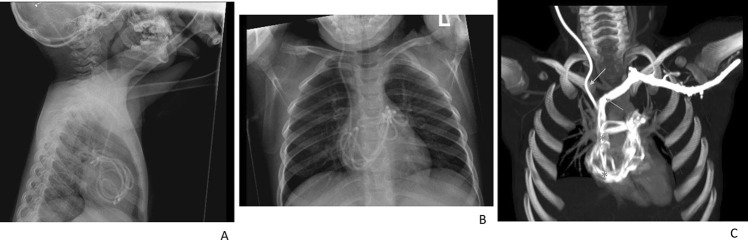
A, B Presenting lateral and AP chest X-rays demonstrating intracardiac positioning and coiling of the distal VP shunt tubing (red stars). C 3D cardiac CT film with contrast demonstrating intracardiac shunt positioning with SVC involvement (yellow arrow), coiling in the right atrium and ventricle (red stars) and extension into both pulmonary arteries (green arrows)
